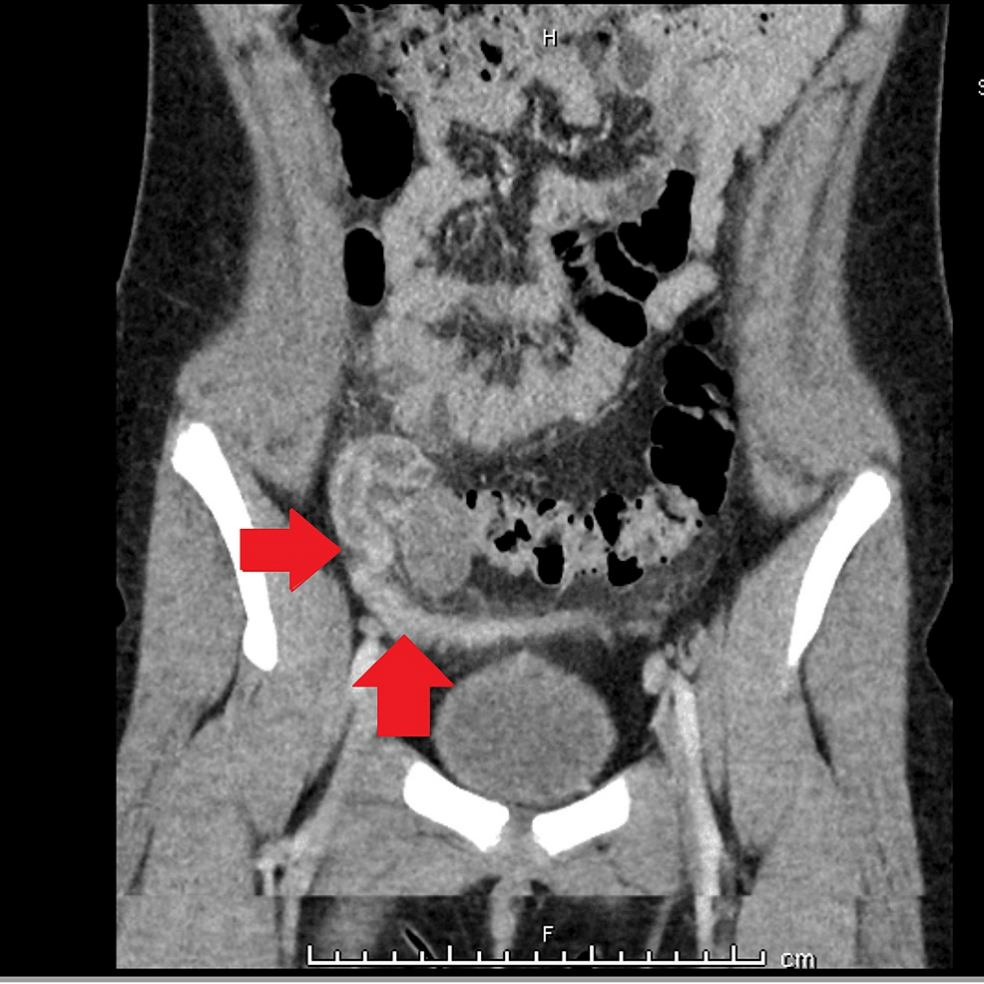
CT abdomen with right fallopian tube with surrounding inflammatory change
